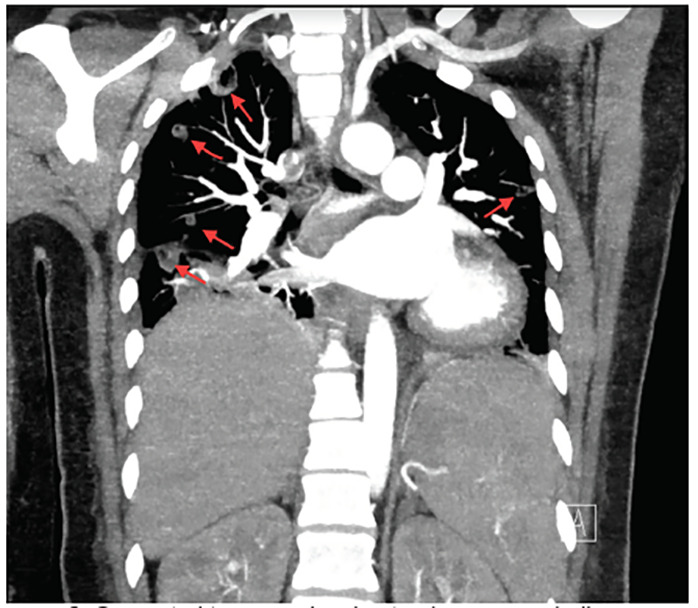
Computed tomography chest pulmonary embolism protocol, demonstrating numerous cavitating nodular consolidations (arrows), concerning for septic emboli.
